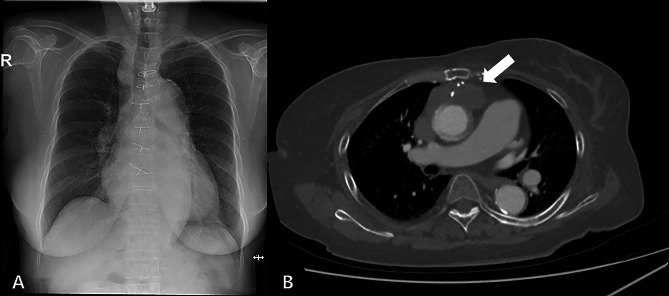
The initial findings on imaging studies. (a) Cardiomegaly was detected on the chest x-ray. (b) Initial chest computed tomography showing a mediastinal mass, possibly a hematoma (white arrow)
